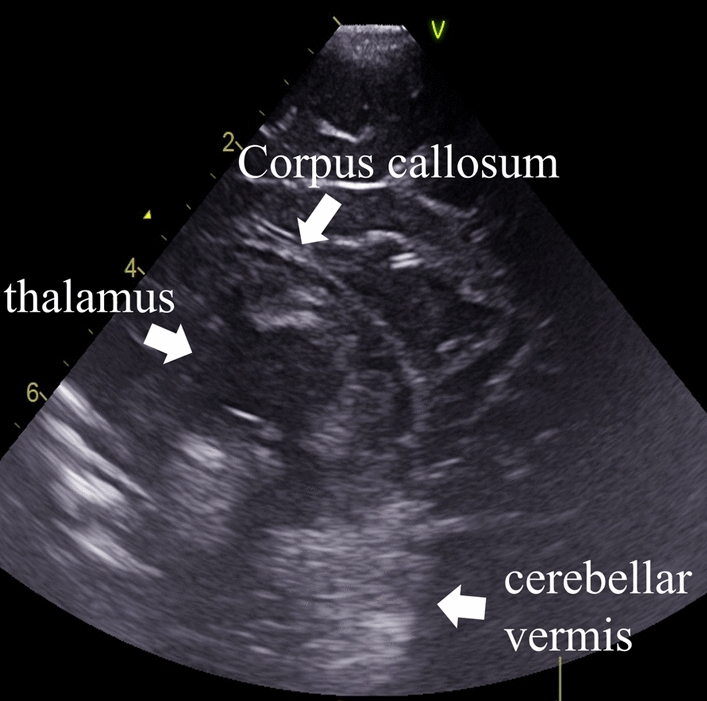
Cranial ultrasound (sagittal view) at 2.5 hours after birth. No hyperechoic lesions indicative of hemorrhage observed. The white arrows indicate normal anatomical structures (corpus callosum, thalamus, and cerebellar vermis) on the cranial ultrasound image
